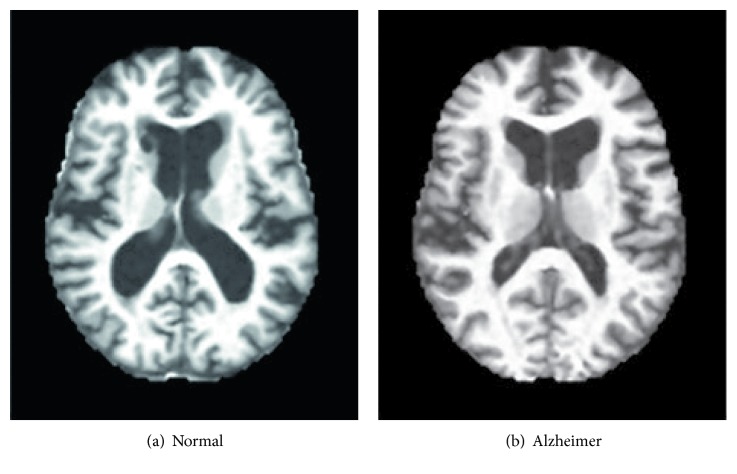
MR image slice sample (axial slice view after preprocessing).
